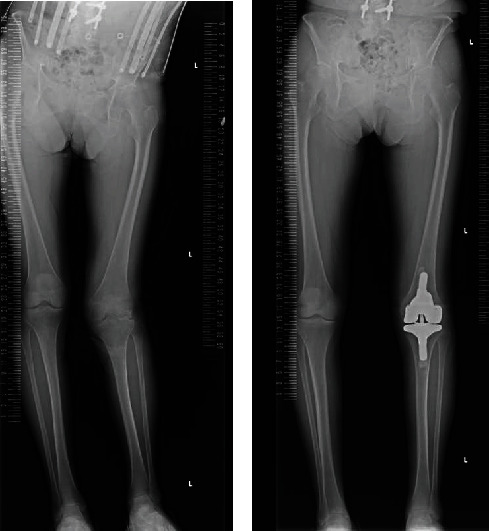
Total alignment of the lower extremity while standing. (a) Radiograph revealing preoperative total alignment of the lower extremity in a standing position. (b) Radiograph revealing postoperative total alignment of the lower extremity in a standing position.
